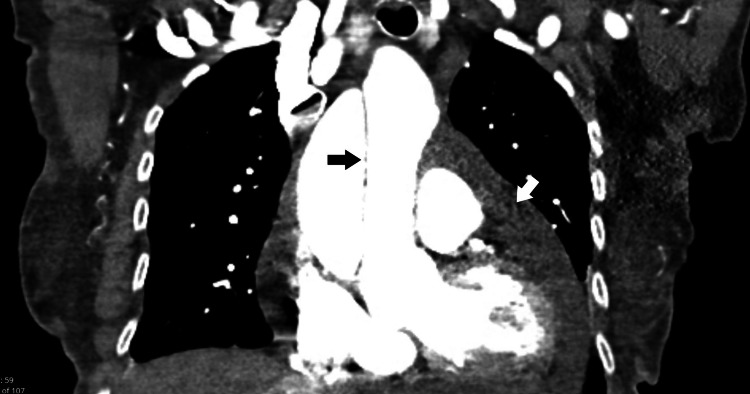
Coronal reconstruction of CTA showing extend of dissection (black arrow) to the aortic arch. Hemopericardium (white arrow) is also seen. CTA: computed tomography angiography
